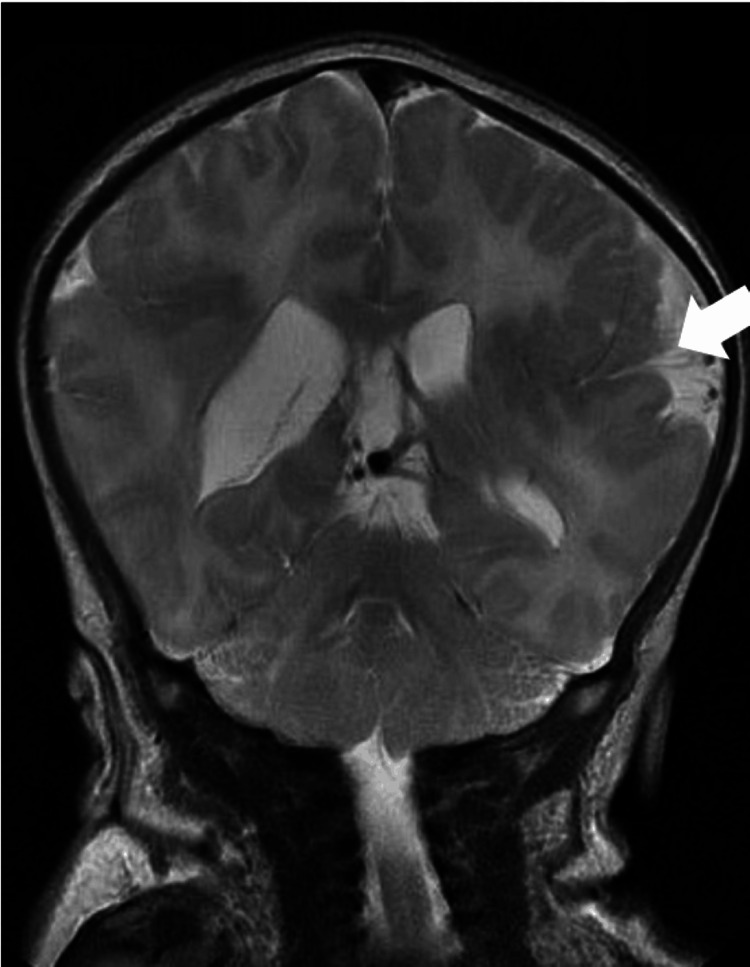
Brain MRI Note polymicrogyria at the frontotemporal junction bilaterally with extension to the insula on the right (white arrow).
